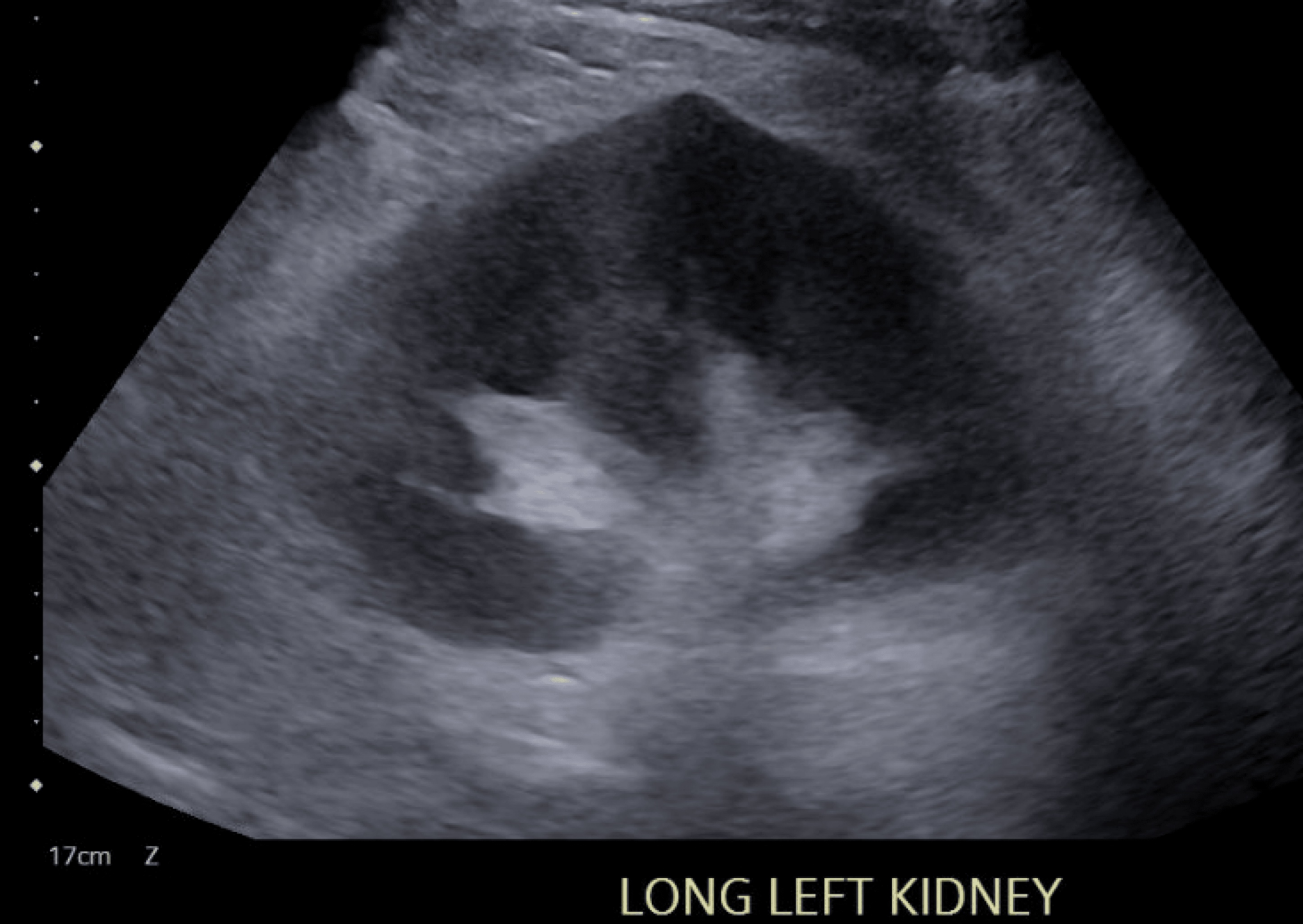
Longitudinal view of the right kidney on renal ultrasound The renal cortex appears homogeneous, the medullary pyramids are distinguishable, and the central echogenic renal sinus is well defined.
